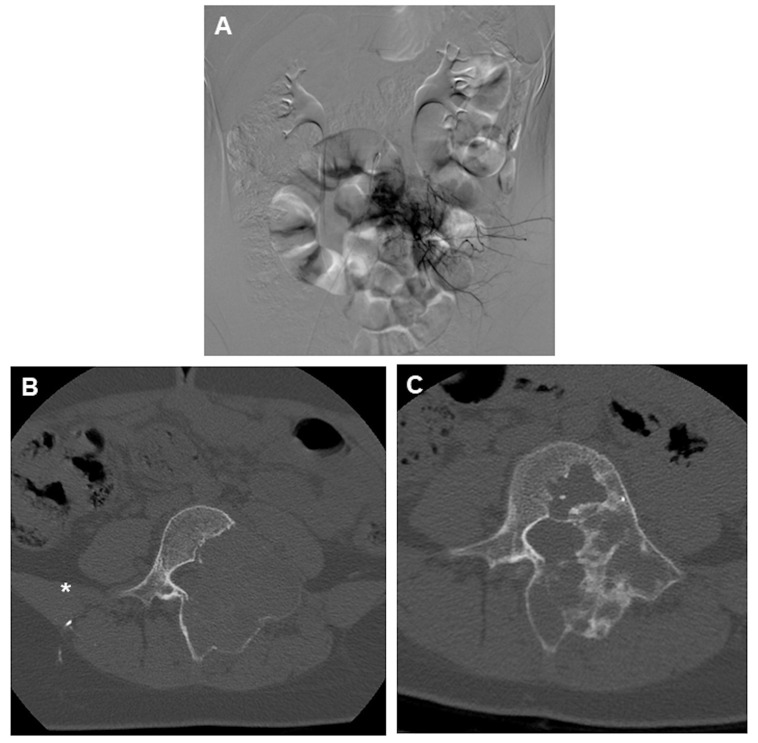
(A) DSA angiogram of an ABC of L4 showing marked hypervascularity of the lesion and (B) the classic appearance of an aneurysmal bone cyst of the spine, with a lytic lesion surrounded by expanded, thin cortical bone. CT was performed right after the embolization, showing the presence of embolic material (asterisk). (C) 1 year follow-up post embolization showing marked recalcification of the lytic lesion.
